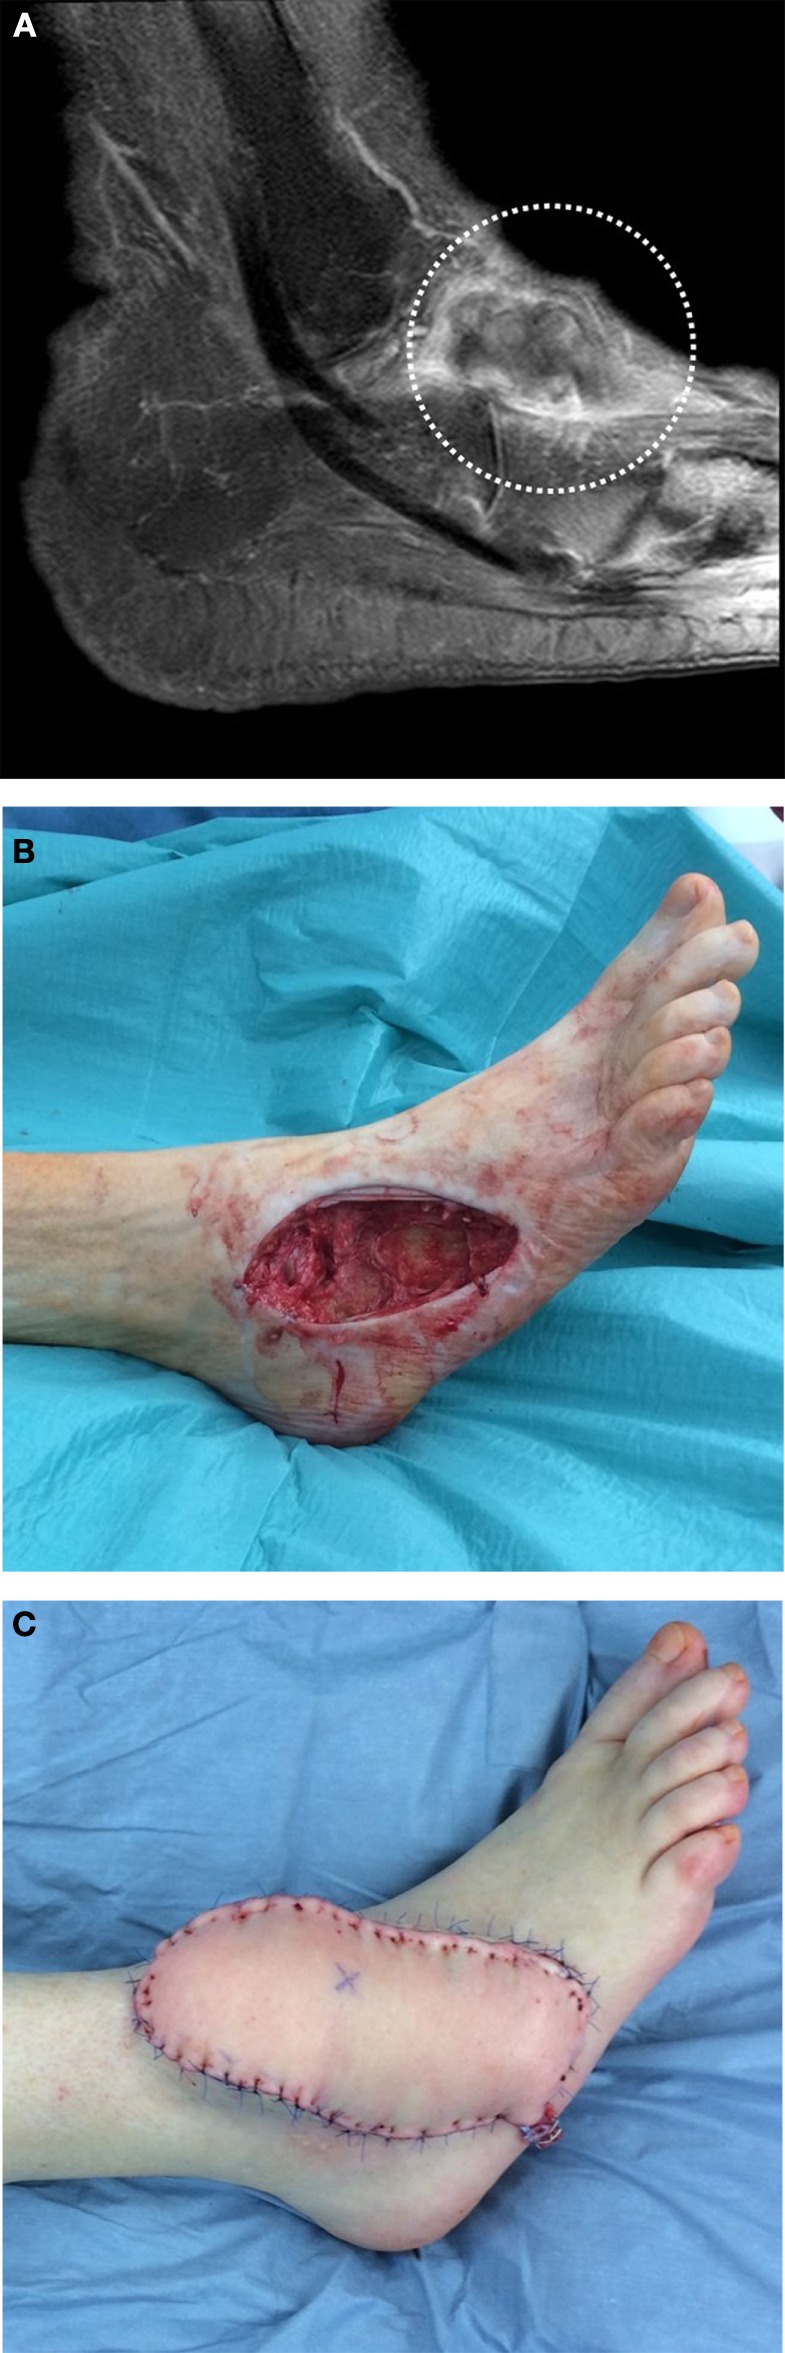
A 77-year-old female was presented after incomplete resection of a liposarcoma G2 on the lateral ankle of her right foot. The initial tumor localization is shown by MRI image (A). A two-stage reconstruction of the defect after extensive resection (B) was done by free transplantation of an adipocutaneous flap (C) from the anterolateral region of the thigh (ALT flap).
